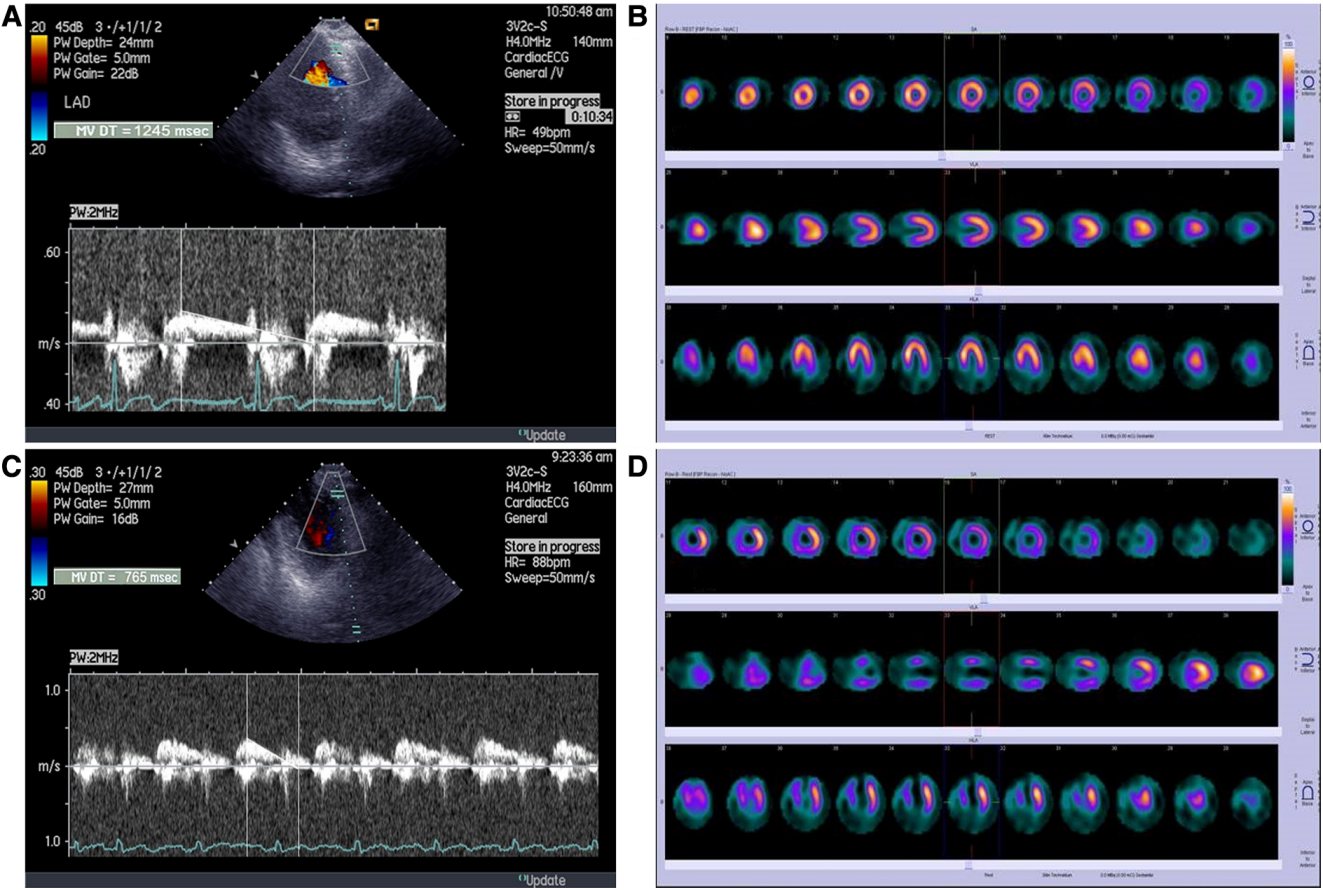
Representative tracings of (A) long DDT of LAD coronary flow velocity B) and SPECT MPI in patient without perfusion abnormalities; (C) short DDT of LAD coronary flow velocity and (D) SPECT MPI in a patient with large fixed perfusion abnormality of 43%.
